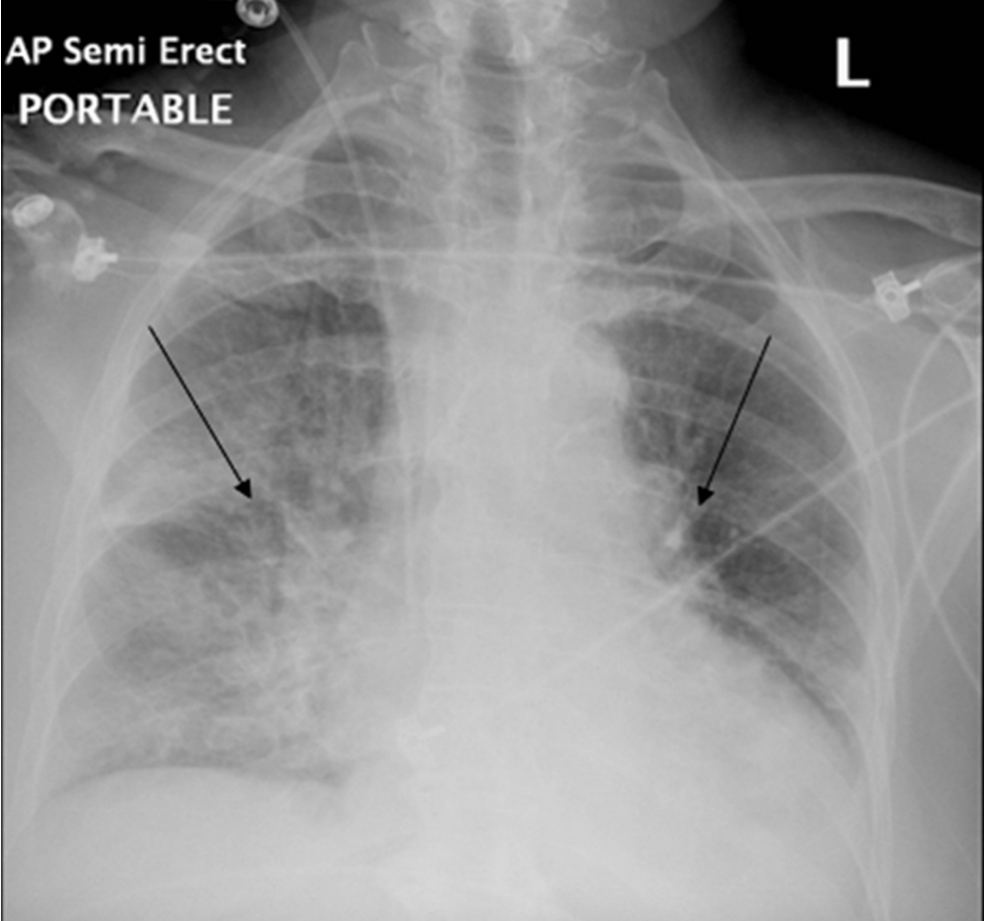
Chest x-ray showing with the black arrows showing vascular congestion/pulmonary edema
